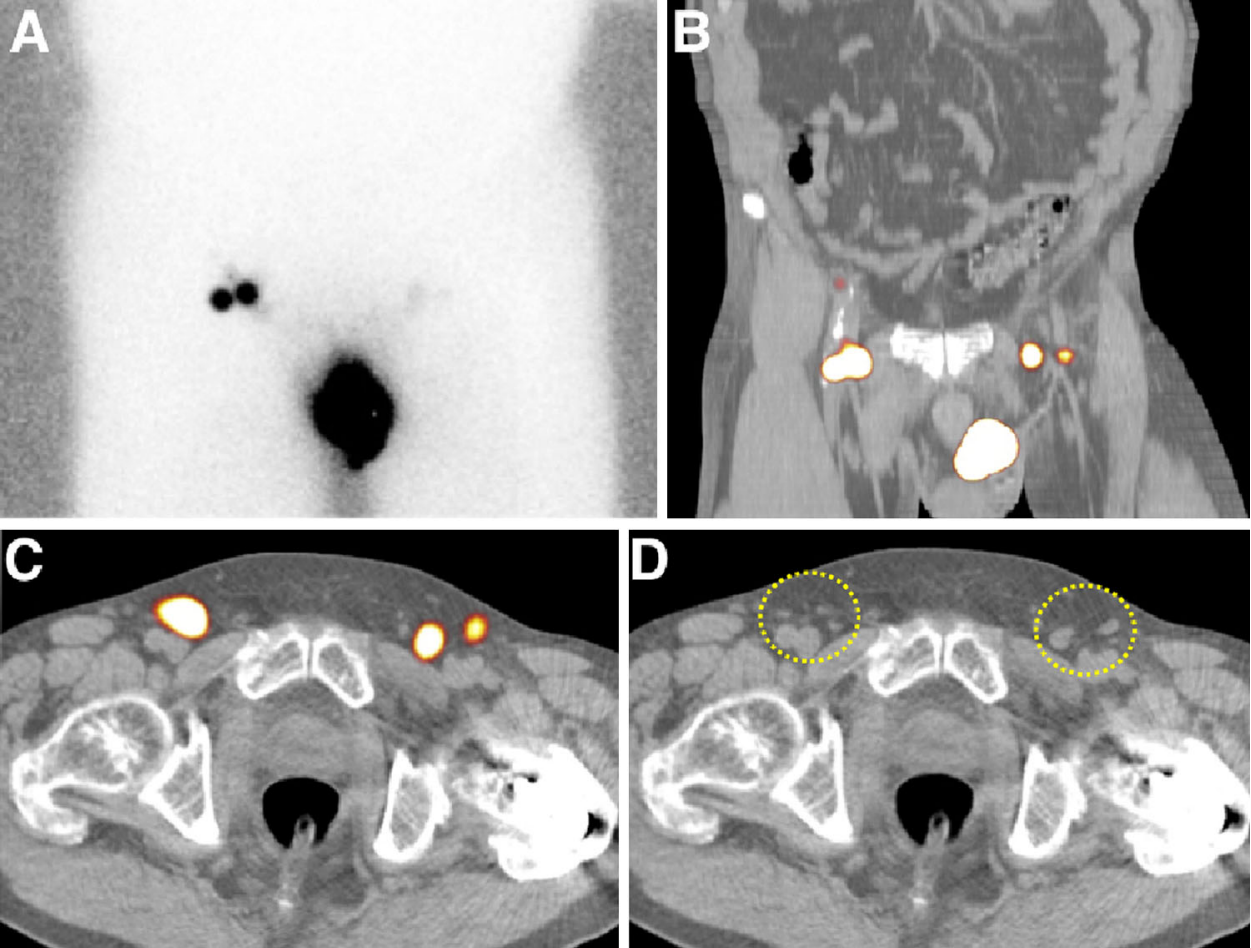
Lymphatic mapping in penile cancer. Planar anterior image a showing drainage to two lymph nodes in the right groin and faint left inguinal uptake. By contrast, coronal (b) and transversal (c) fused SPECT/CT show clear uptake in the left groin corresponding to two lymph nodes (*circle*) along both sides of the femoral vessels as seen on CT (d). Note that intense uptake in the right groin corresponds with a cluster of four lymph nodes (*circle*)
